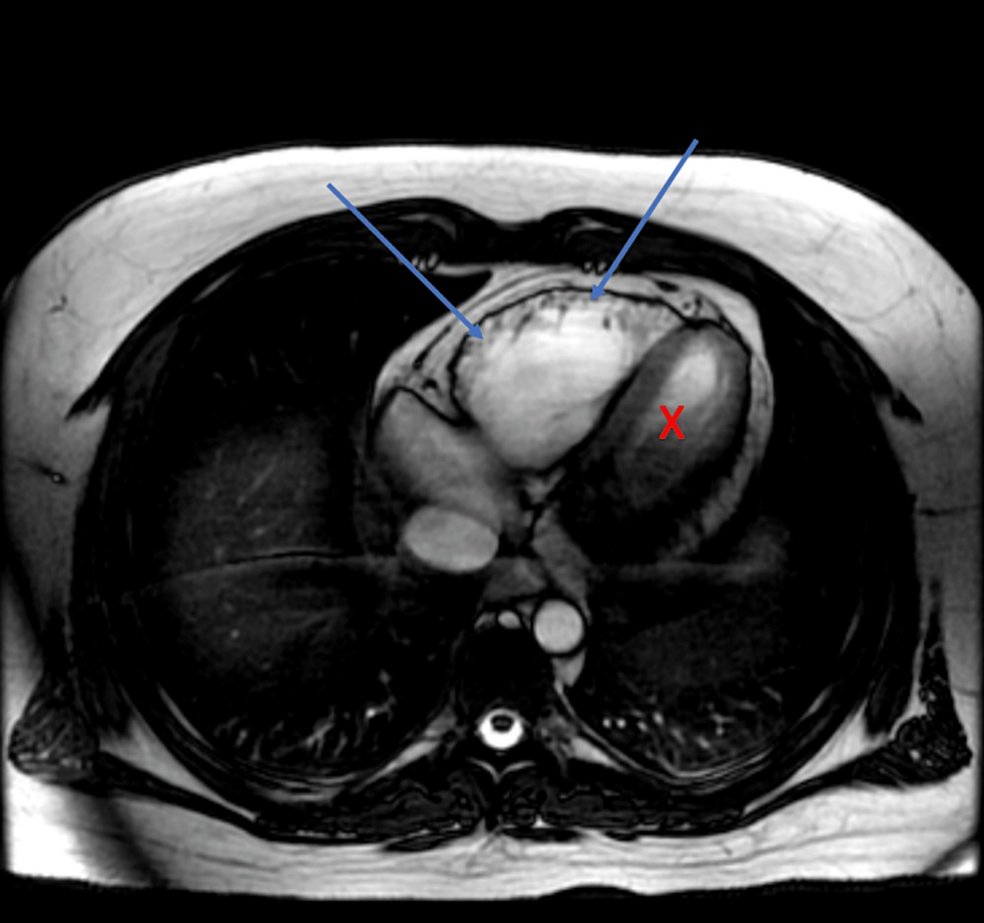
Cardiac MRI with blue arrows indicating right ventricular dilation consistent with ARVC. “X” denotes the left ventricle.
